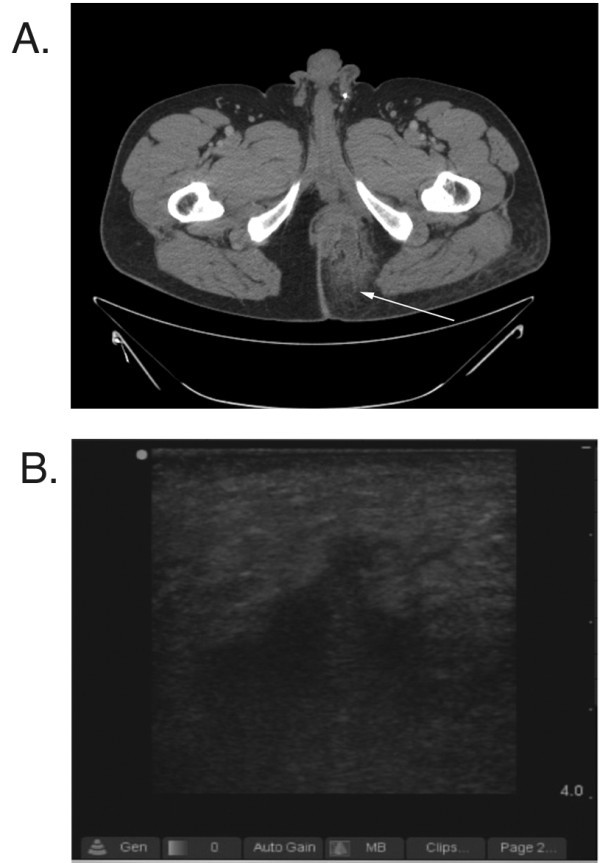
US and CT of patient with perirectal abscess. Figure (A) demonstrates CT imaging of a patient with a perirectal abscess. Note the stranding in the soft tissue (white arrow) but lack of visualized abscess cavity as interpreted by the original radiologist, and (B) demonstrates sonographic imaging of the same patient (long axis orientation) with characteristic loss of soft tissue planes superior and lateral to an anechoic abscess cavity. An extension of the abscess cavity "points" towards the skin surface.
